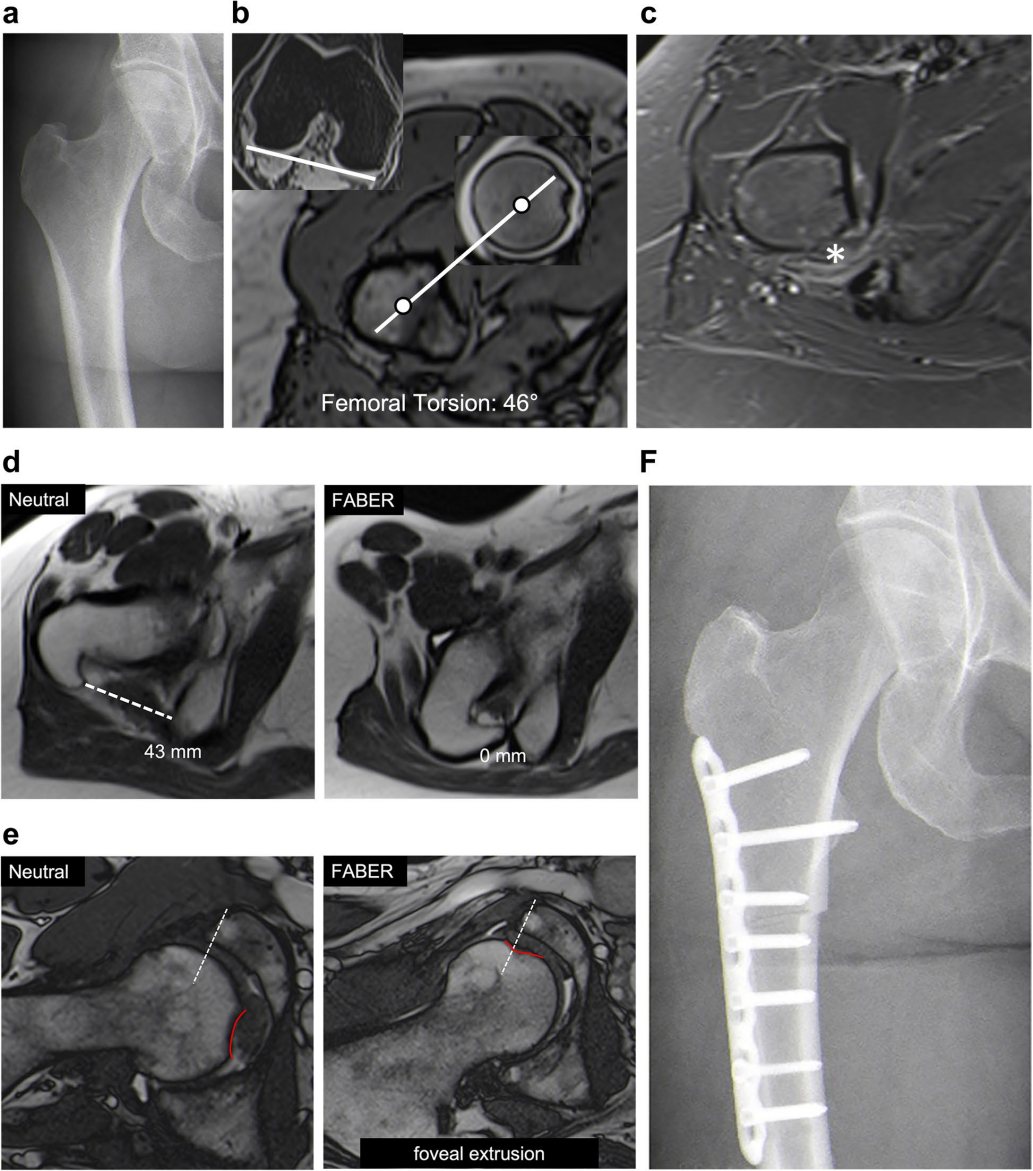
a Anteroposterior radiograph of the right hip of a patient with (b) increased femoral torsion of 46° according to Murphy and a (c) quadratus femoris muscle edema on axial STIR images. FABER-MRI revealed a reduction in the (d) proximal intertrochanteric distance from the neutral to the FABER position, in which (e) furthermore, a foveal excursion was evident. f Postoperative radiograph after subtrochanteric derotation osteotomy
